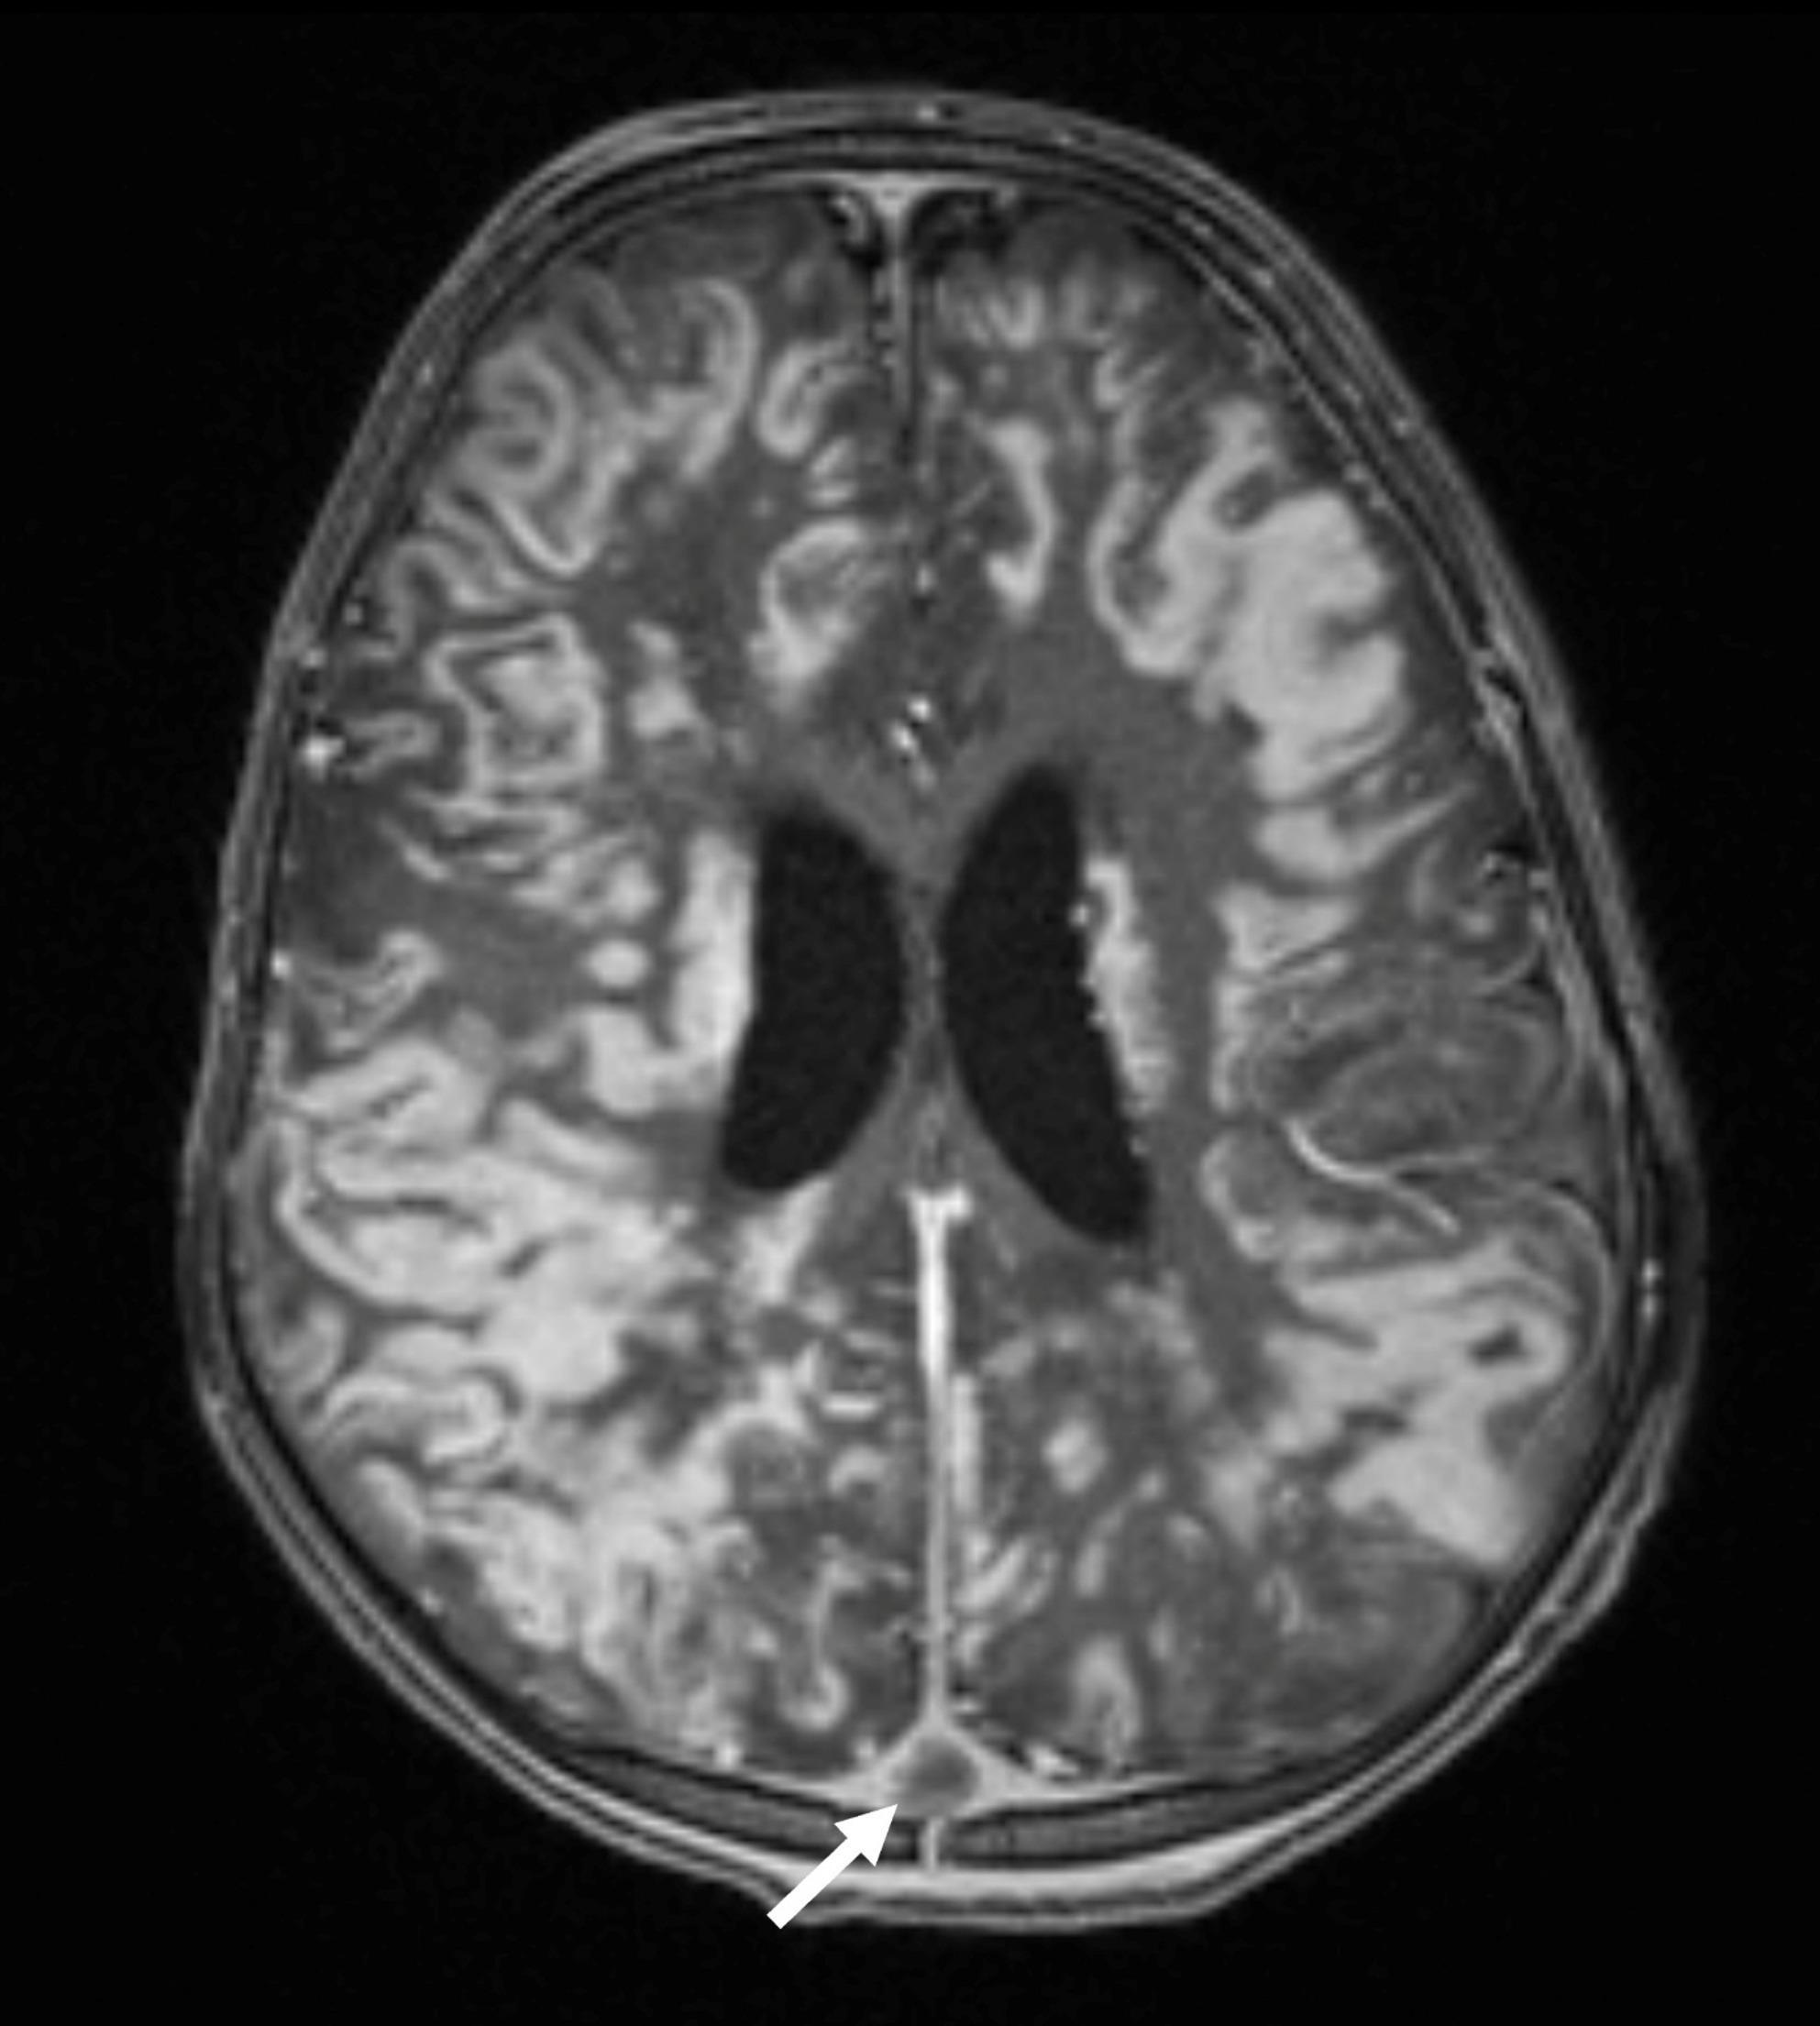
Contrast-enhanced T1-weighted image at the supratentorial level demonstrates diffuse enhancement in the cortical and subcortical regions, along with involvement of the corpus callosum. In addition, thrombosis is observed in the superior sagittal vein (arrow)
